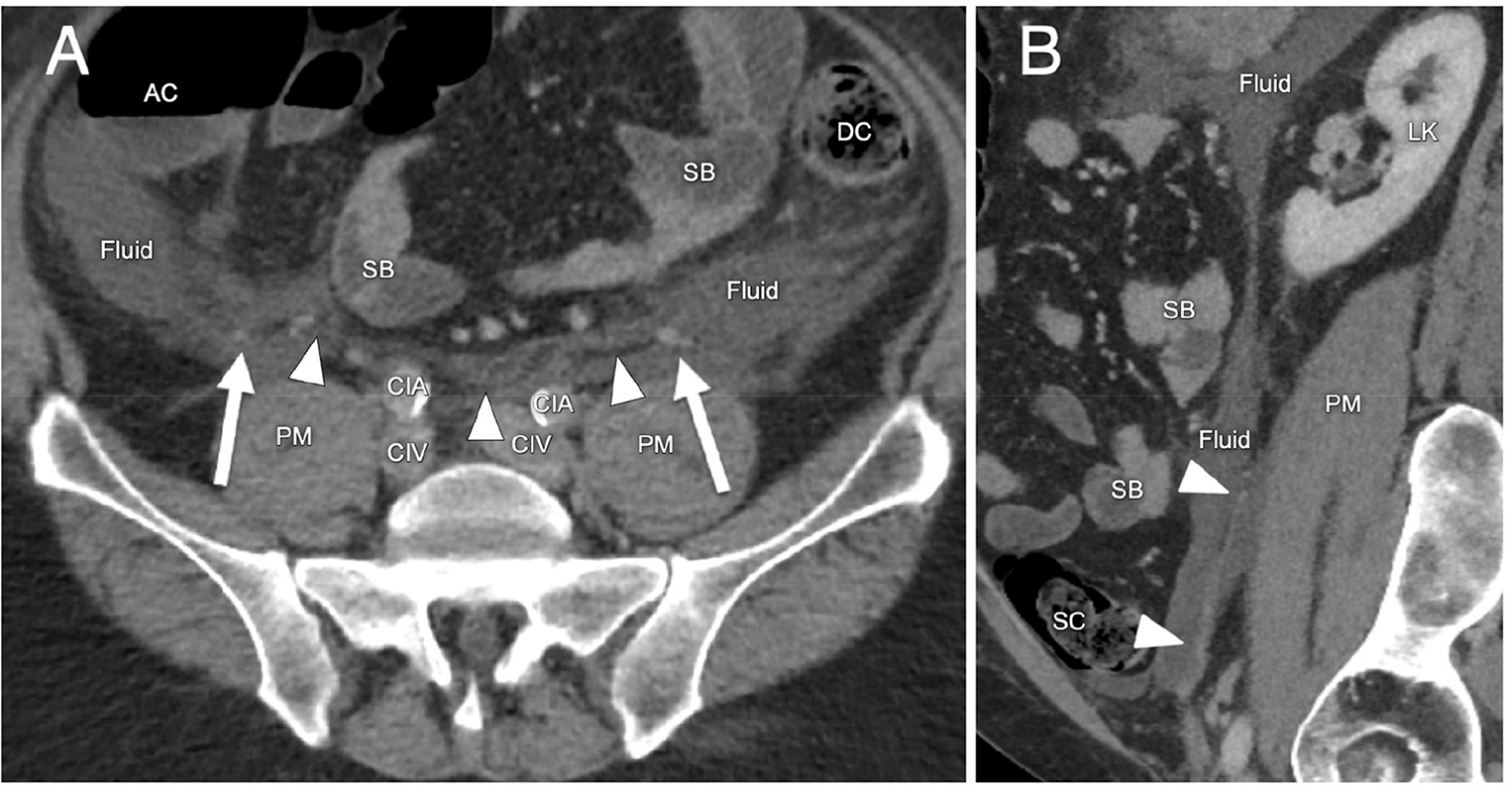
Retroperitoneal fluid in a patient with pancreatitis demonstrated on CT imaging. A) At the level of the pelvis fluid tracks around the caudal extension of the renal fascia which is visible as a bilateral sheath of adipose tissue. The gonadal vessels (arrows), mark the approximate lateral border of the caudal extension of the renal fascia. B) Sagittal reconstructions demonstrate the caudal extension of the renal fascia (arrow heads), containing the ureter and testicular vessels coursing to the inguinal canal. AC: ascending colon, CIA: common iliac artery, CIV: common iliac vein, DC: descending colon, LK: left kidney, PM: psoas major muscle, SB: small bowel, SC: sigmoid colon
